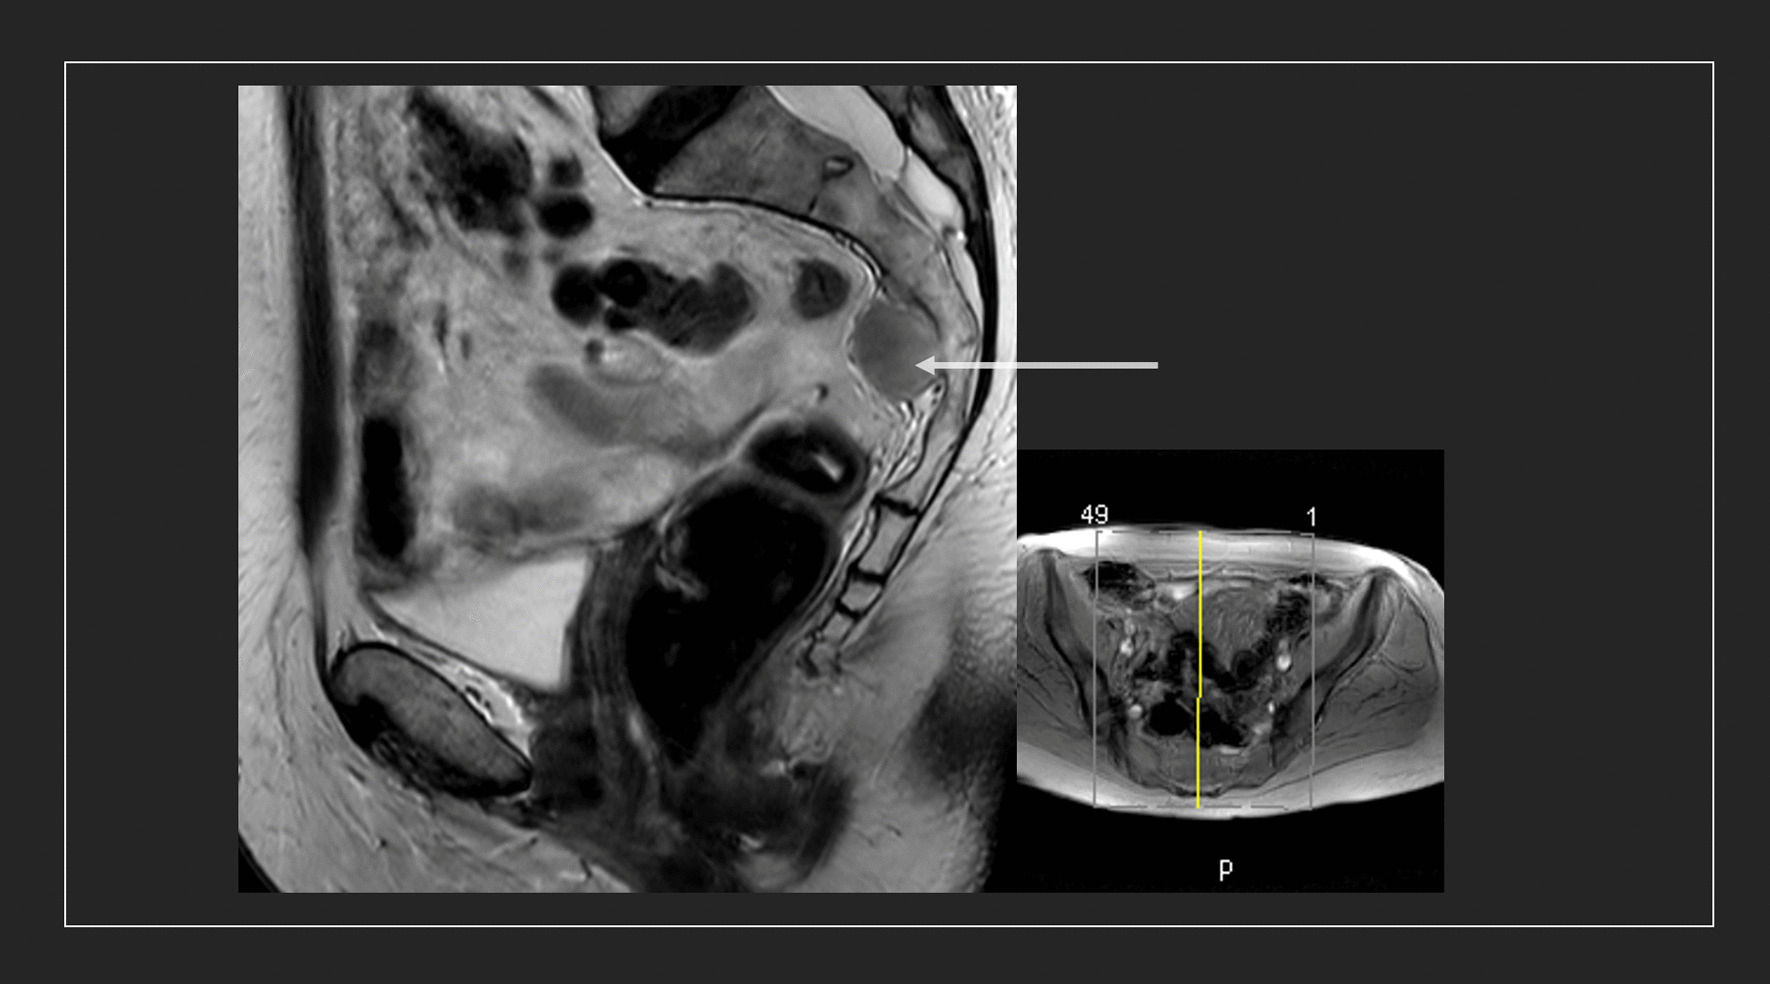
A 71-year-old female. T2-weighted (TSE) MRI scan (sagittal) of presacral myelolipoma (arrow). Image shows an incidental heterogeneous presacral tumor at the level of S3
